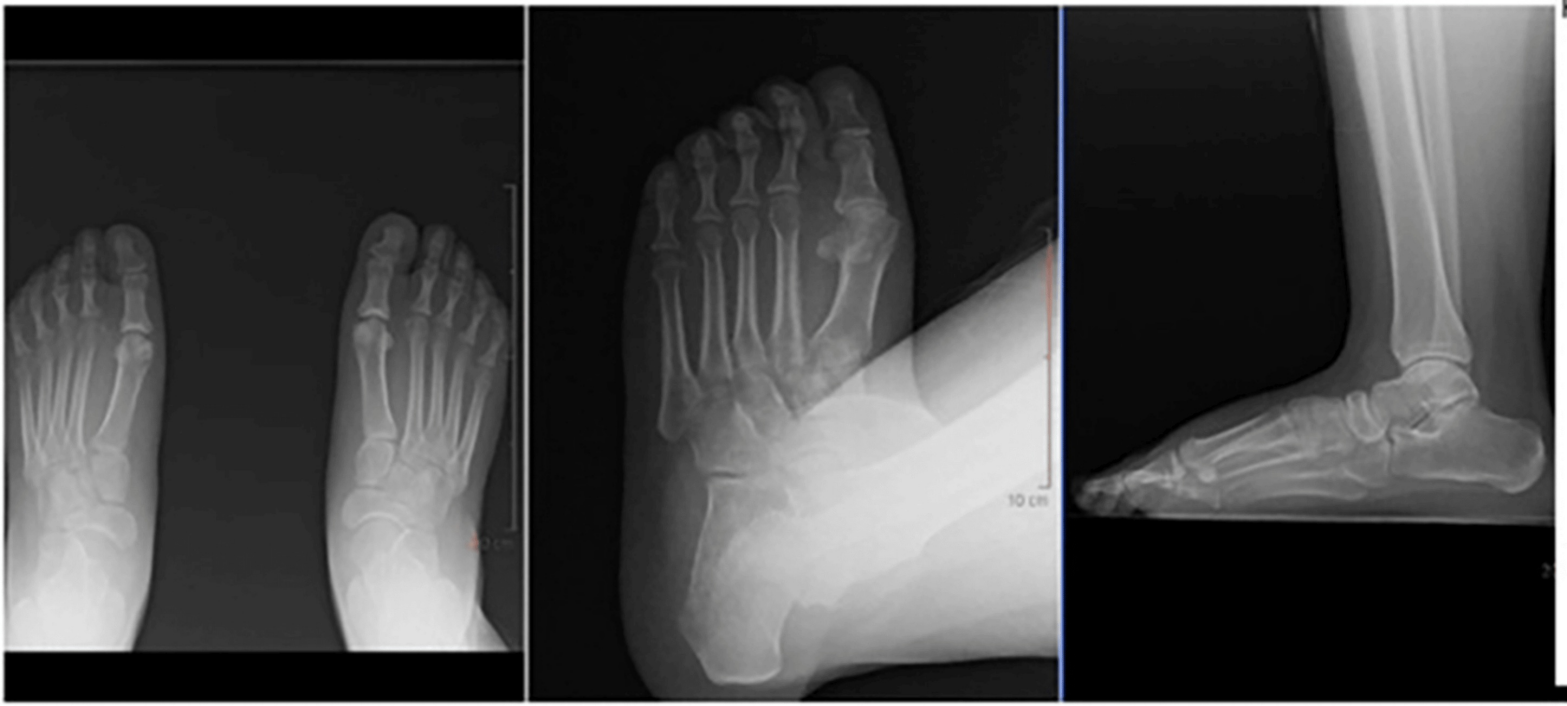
Example of mock patient scenario (Case 5) provided to surgeons; Pre-operative radiographs demonstrating subacute Lisfranc injury and 2nd tarsometatarsal joint dislocation.
